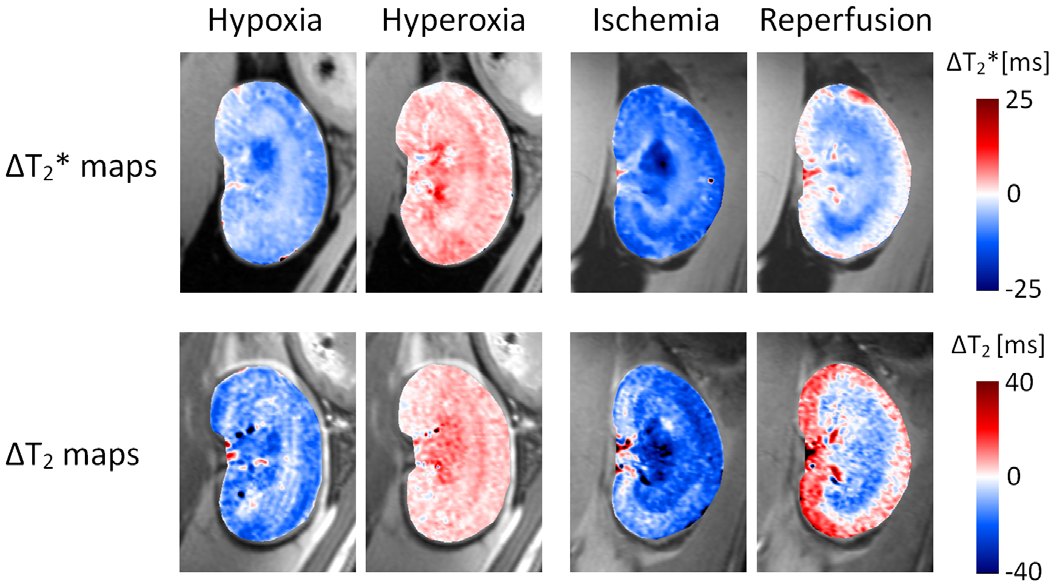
Change of renal T_2_* and T_2_ during hypoxia, hyperoxia, ischemia and reperfusion. Shown are T_2_* and T_2_ difference maps of the kidney (color-coded, overlay on anatomical MR image) between the last time point in each experiment phase and baseline. The three interventions (hypoxia, hyperoxia, ischemia) led to a reasonably homogeneous decrease/increase in both parameters. During ischemia the magnitude of the T_2_*/T_2_ reduction exceeded that during hypoxia. Parameter changes in the reperfusion phase clearly differentiated cortex and medulla: while medullary T_2_*/T_2_ remained reduced throughout reperfusion, in the cortex T_2_* returned to baseline and T_2_ rose above baseline.
